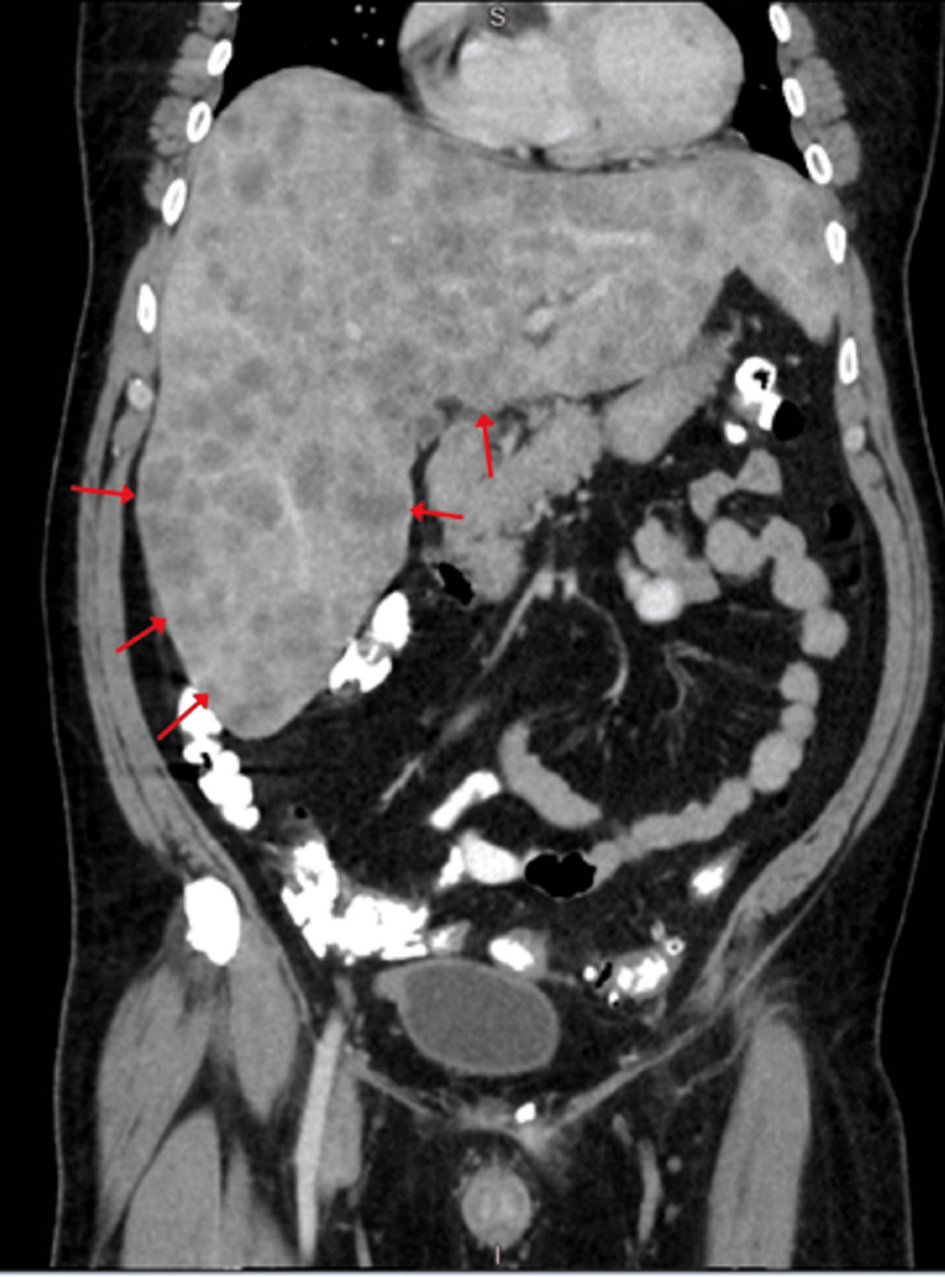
CT Abdomen/Pelvis with contrast showing multiple liver metastases (red arrows)
